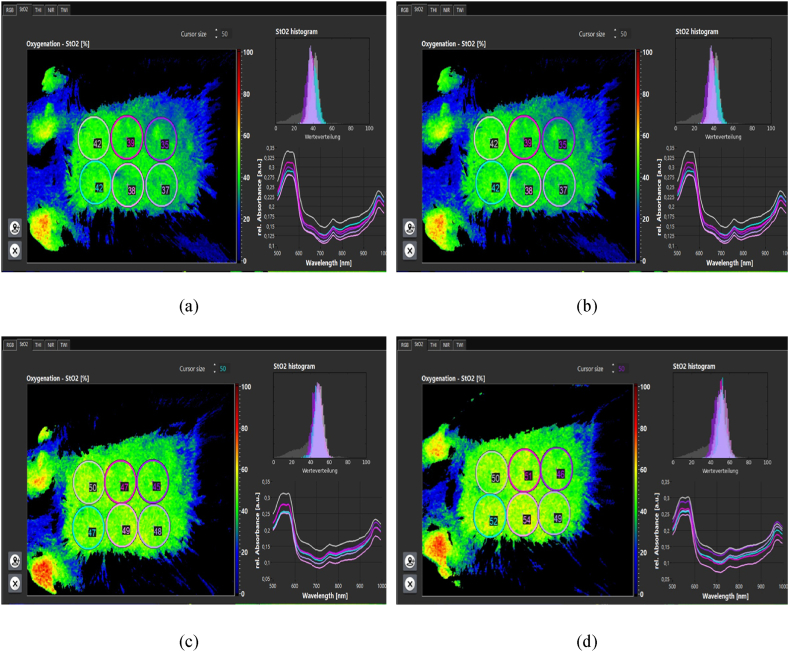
Ultrasound images of the plasma group. (a) Before treatment. (b) Immediately after treatment. (c) Forth week. (d) Tenth week.
